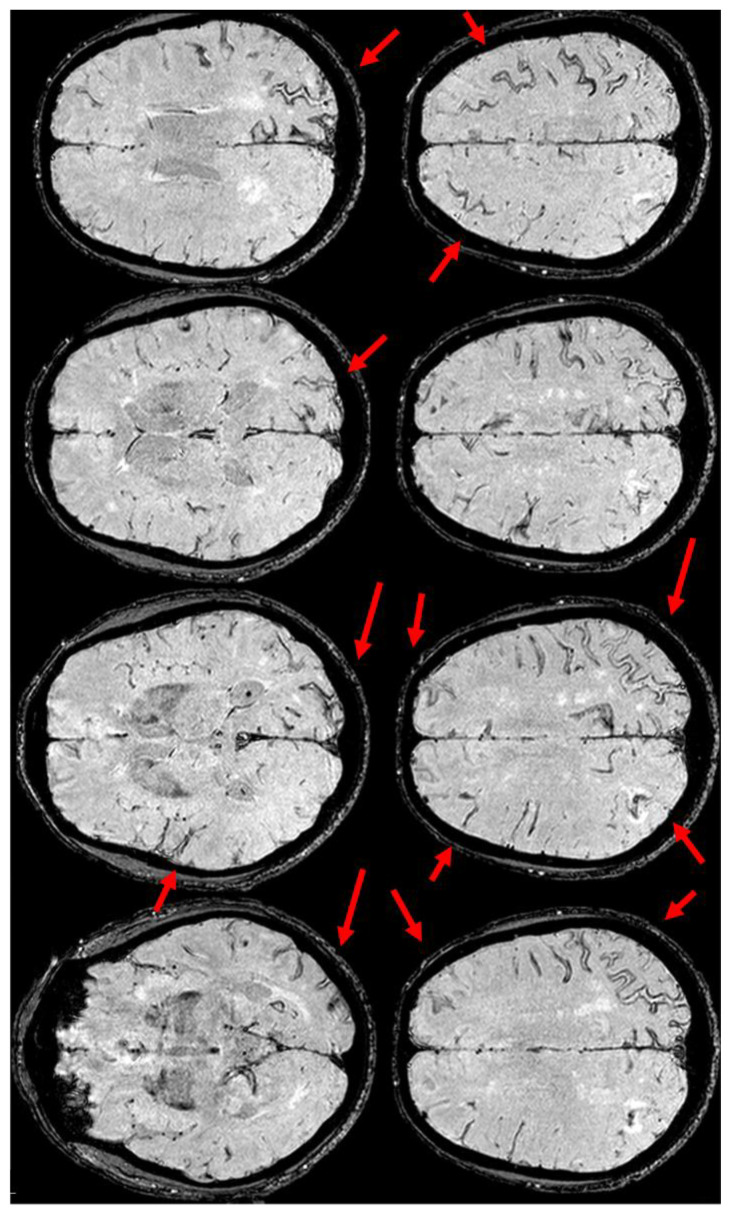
Brain MRI (Susceptibility Weighted Imaging—SWI) in the axial plane, showing the extension of cSS to the left posterior temporal and occipital lobe, to the left posterior parietal lobe, to the parasagittal sulci at the vertex on both sides, and to the right frontal and parietal lobes (red arrows).
